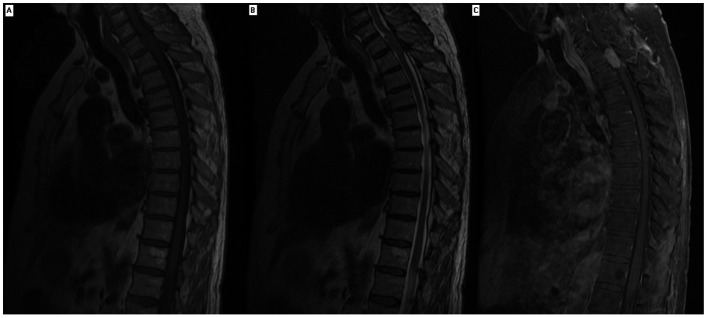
Magnetic resonance imaging of thoracic spine of a patient with paraplegia. Contrast enhancing magnetic resonance imaging showed solid homogenous intradural extramedullary mass extends from level of first thoracic vertebrae superior endplate to superior aspect of second thoracic vertebrae resulting in spinal cord compression. The mass was isointense on MRI T1 and T2 sequence.
